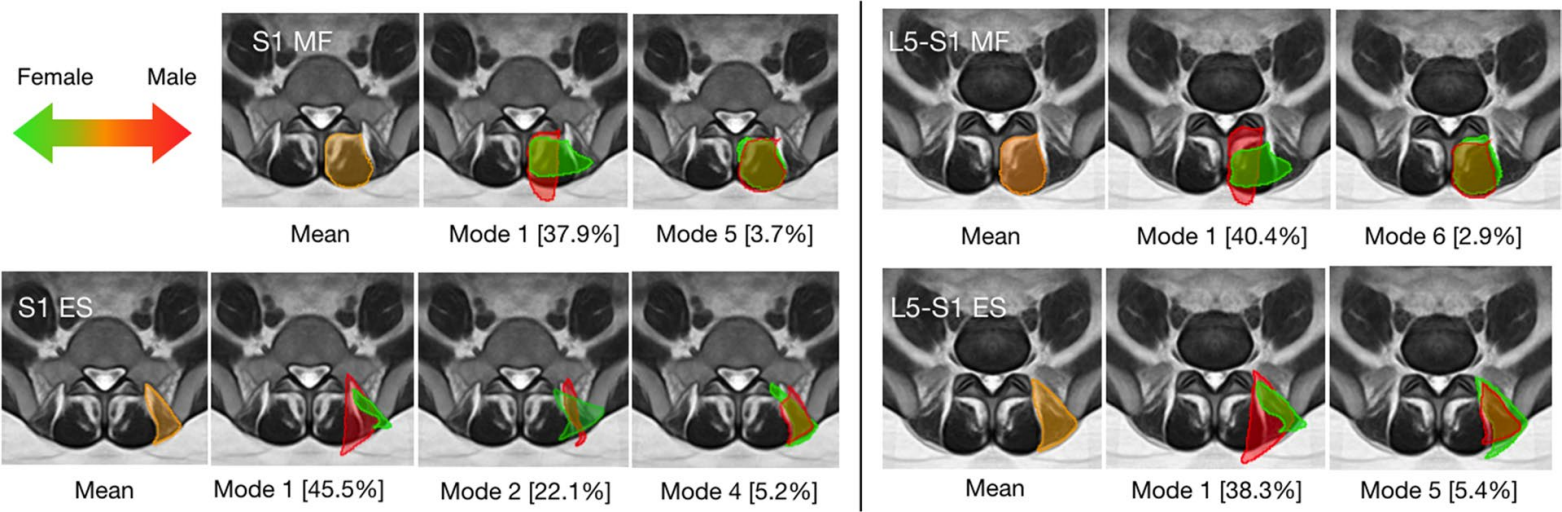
Modes of the multifidus (MF) and erector spinae (ES) shape associated with sex. The mean shape is shown in orange overlaid on population-averaged MRI templates. The trend of shape variation towards female sex is in green and the opposite is in red. For each mode, threefold standard deviations from the mean shape are depicted for both directions of shape variants, and the percentages of shape variance explained are included in [ ] for all modes.
